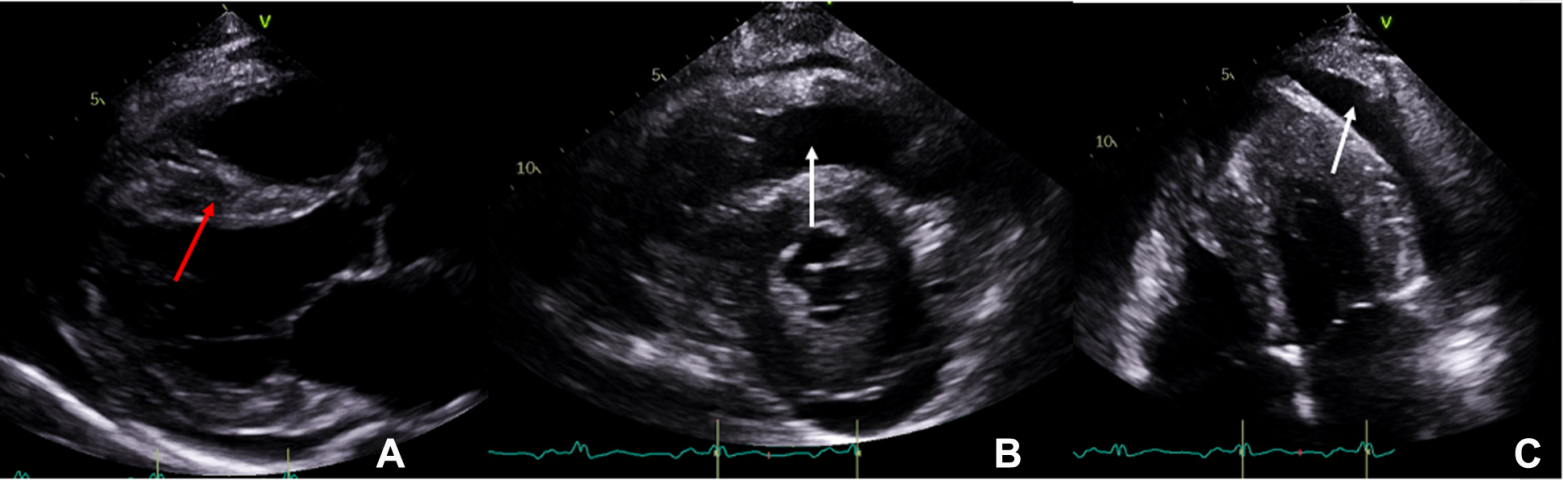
Initial Transthoracic Echocardiogram Initial transthoracic echocardiogram demonstrating a small pericardial effusion, 1.8 cm in maximum diameter next to the lateral left ventricle wall but 1.2 cm elsewhere (white arrows) and concentric mild left ventricular hypertrophy toward the mid-ventricle and apex (red arrow, foreshortened windows). (A) Parasternal long-axis view. (B) Parasternal short-axis view. (C) Apical 4-chamber view.
